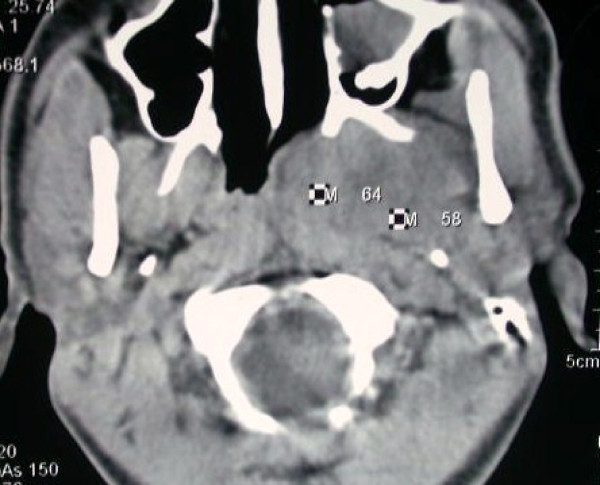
Axial CT scan showing homogenously enhancing lesion.
